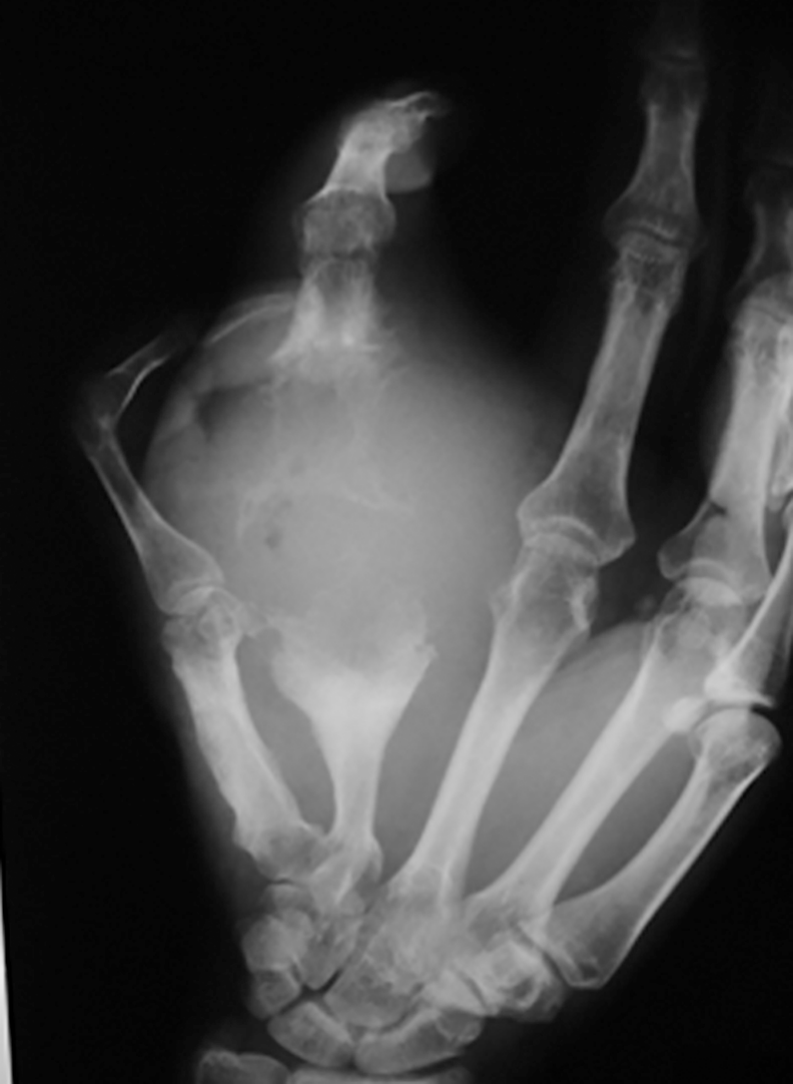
X-ray of right hand showing massive soft tissue mass, periosteal reaction and bone cavities in line with eumycetoma appearance.
